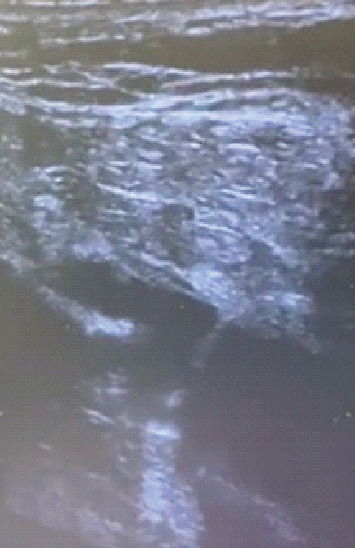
Ultrasound-guided drainage of the right shoulder's abscess.
